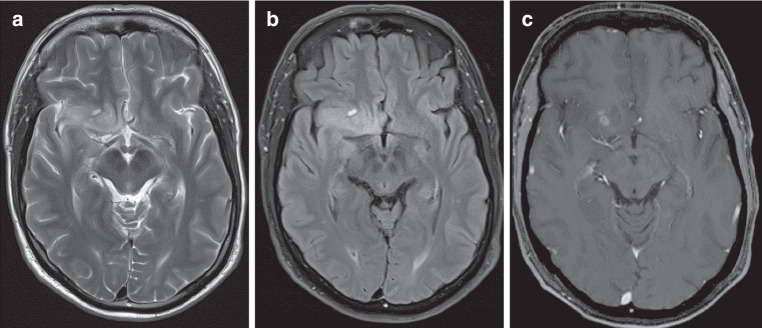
a–c Glioblastoma without mutation of isocitrate dehydrogenase (IDH-wildtype), with amplification of the gene for the epidermal growth factor receptor (EGFR amplification), gain of chromosome 7 and loss of chromosome 10, World Health Organization grade 4 (WHO grade 4) (male, age: 52 years) temporo-insular and frontobasal right with only small areas of contrast enhancement. a T2-weighted image, b fluid attenuation inversion recovery MR sequence (FLAIR), c T1-weighted image after i.v. application of contrast agent. Presence of EGFR amplification or telomerase reverse transcriptase promotor mutation (TERT promotor mutation) or chromosome 7+/10− copy number alterations or any combination of these features in a diffuse astrocytic glioma IDH-wildtype/H3-wildtype is diagnostic for a glioblastoma WHO grade 4, even if there is no obvious radiological sign of a glioblastoma such as necrosis
